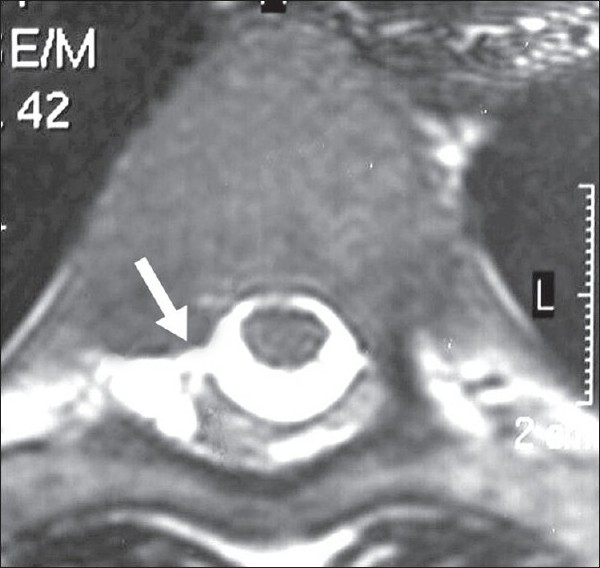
Axial T2 weighted MRI spine at T6 vertebral level showing a right paraspinal extra-arachnoid CSF collection with a communicating tract (arrow) to subarachnoid CSF (Case 1)
